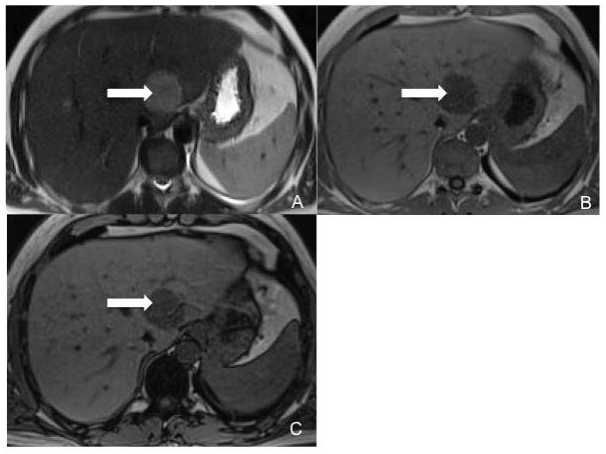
T2-weighted HASTE sequence on axial plane. Shows one of the solid liver lesions in the II segment, with the same patterns of signal intensity showed by the mediastinal mass on the (A) T2-weighted and (B,C) T1-weighted images before contrast median injection (white arrows).
